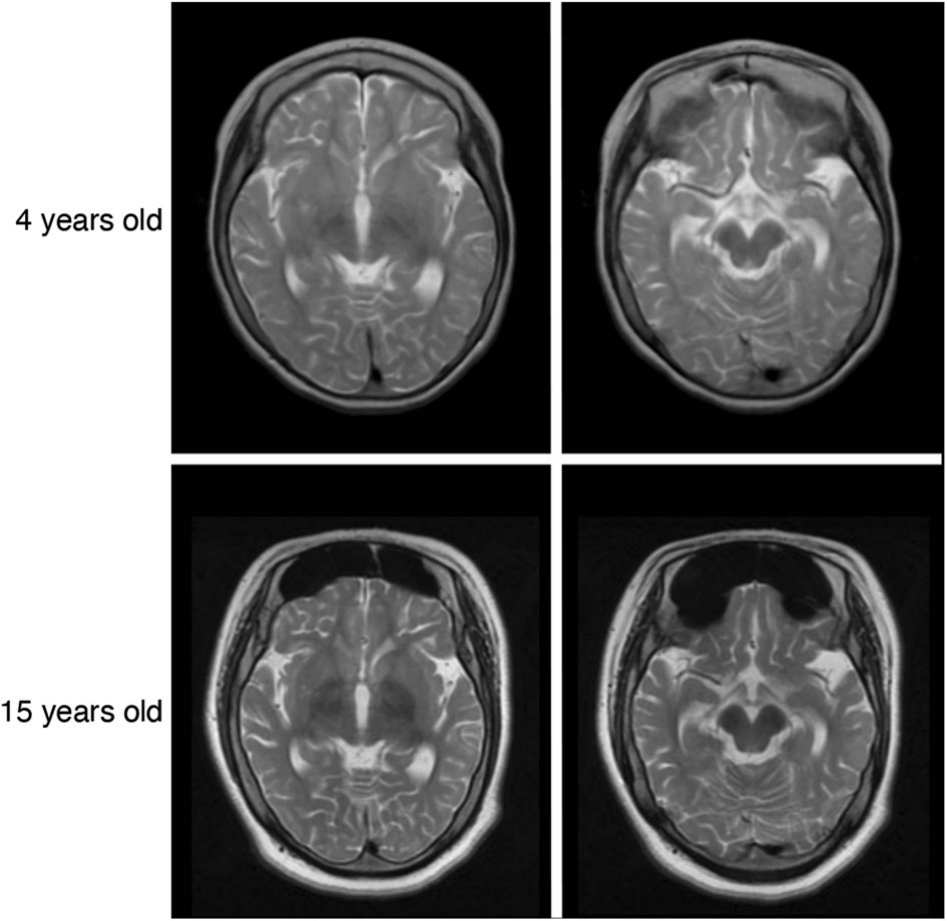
MRI at the age of 4 and 15 years. Top: MRI at the age of 4 years showed very little iron deposition in the globus pallidus (Left) and substantia nigra (Right) on T2 MRI. Bottom: MRI at the age of 15 years showed the appearance of iron deposition in the globus pallidus (Left) and substantia nigra (Right) on T2 MRI.
